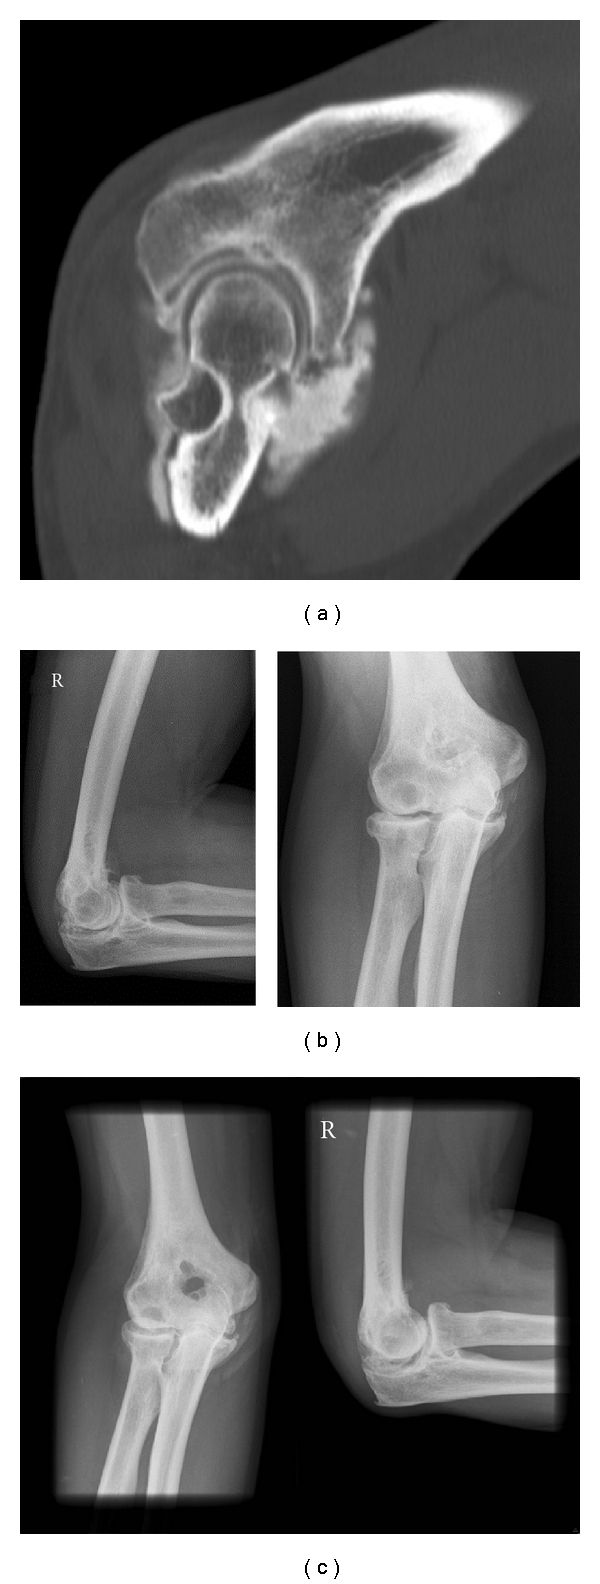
Radiological assessment with CT scan of early cubarthritis shows the posterior impingement in extension (a). Pre- (b) and postoperative (c) X-rays of the perforation of the distal humerus.
